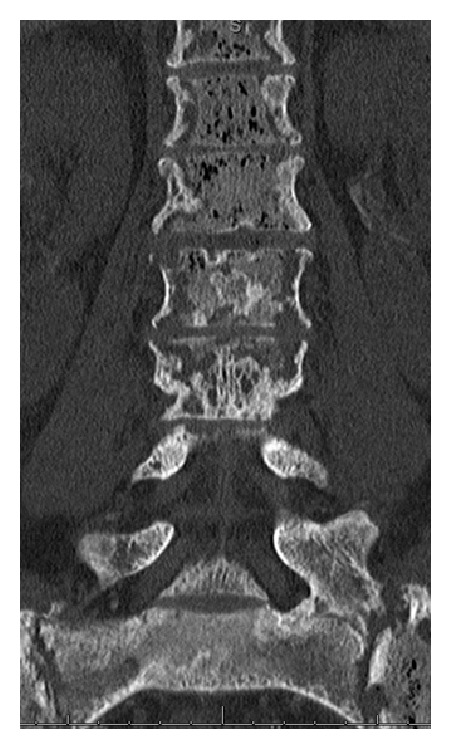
CT lumbar spine without contrast approximately 8 months since the initial presentation shows continued progression of diffuse osteonecrosis of the visualized marrow. The bone marrow is abnormal with multiple foci of gas.
